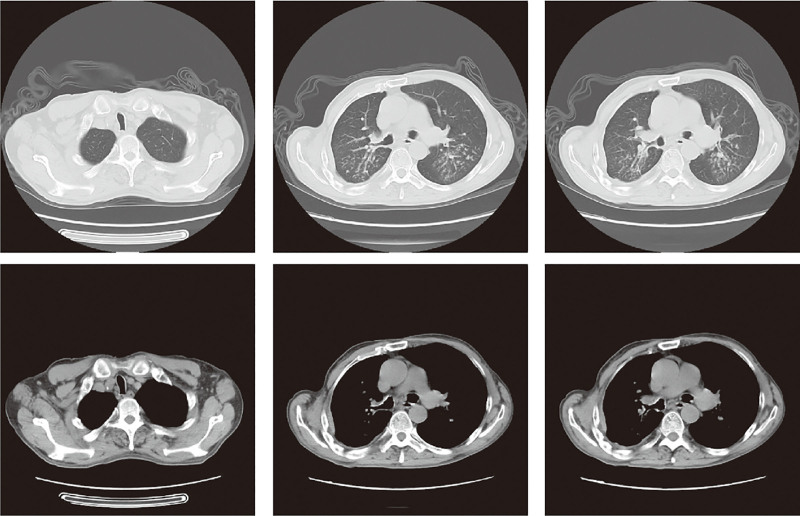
Chest CT showing both lungs show blurred texture enhancement. Multiple patchy opacities distributed along bronchial courses are visible in both lungs. Multiple bronchial walls are thickened with calcification. Partial atelectasis is noted in the middle and lower lobes of the right lung and the lower lobe of the left lung. No significantly enlarged mediastinal lymph nodes are visible. The thoracic cage is symmetrical. No definite pleural effusion is visible within the thoracic cavity. Diffuse thickening of the right pleura is present. CT = computed tomography.
